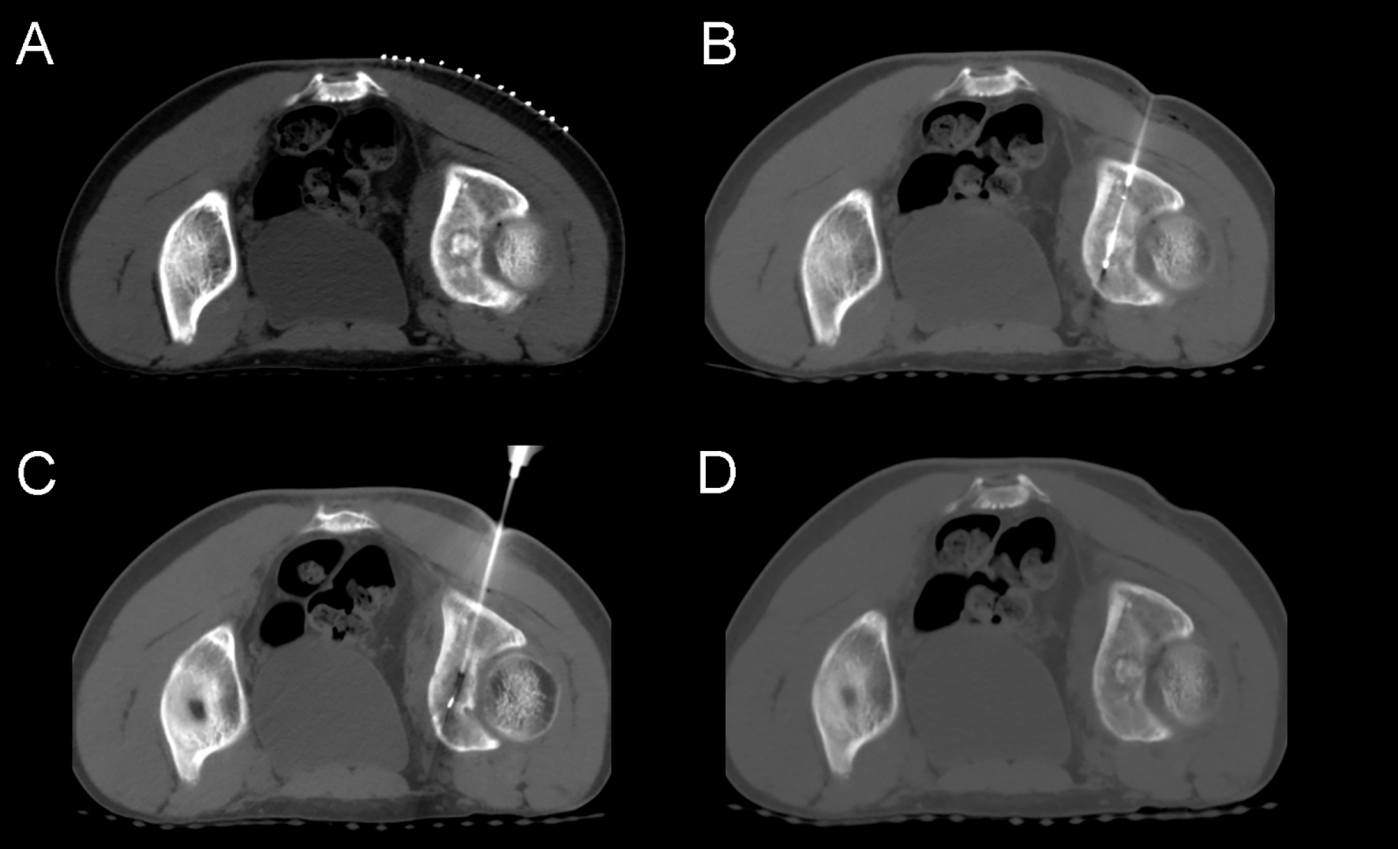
A 15-year-old male patient with left hip pain and claudication. (A) A large, round, high-density lesion was seen in the left acetabulum, surrounded by a circular hypodensity shadow. (B) Cryoprobe passed anteriorly through the lesion. (C) After two cycles of freezing, the lesion did not change significantly on CT images. No obvious ice ball could be seen. (D) After removal of the needle, the case was reviewed, and no other complications such as bleeding were found.
